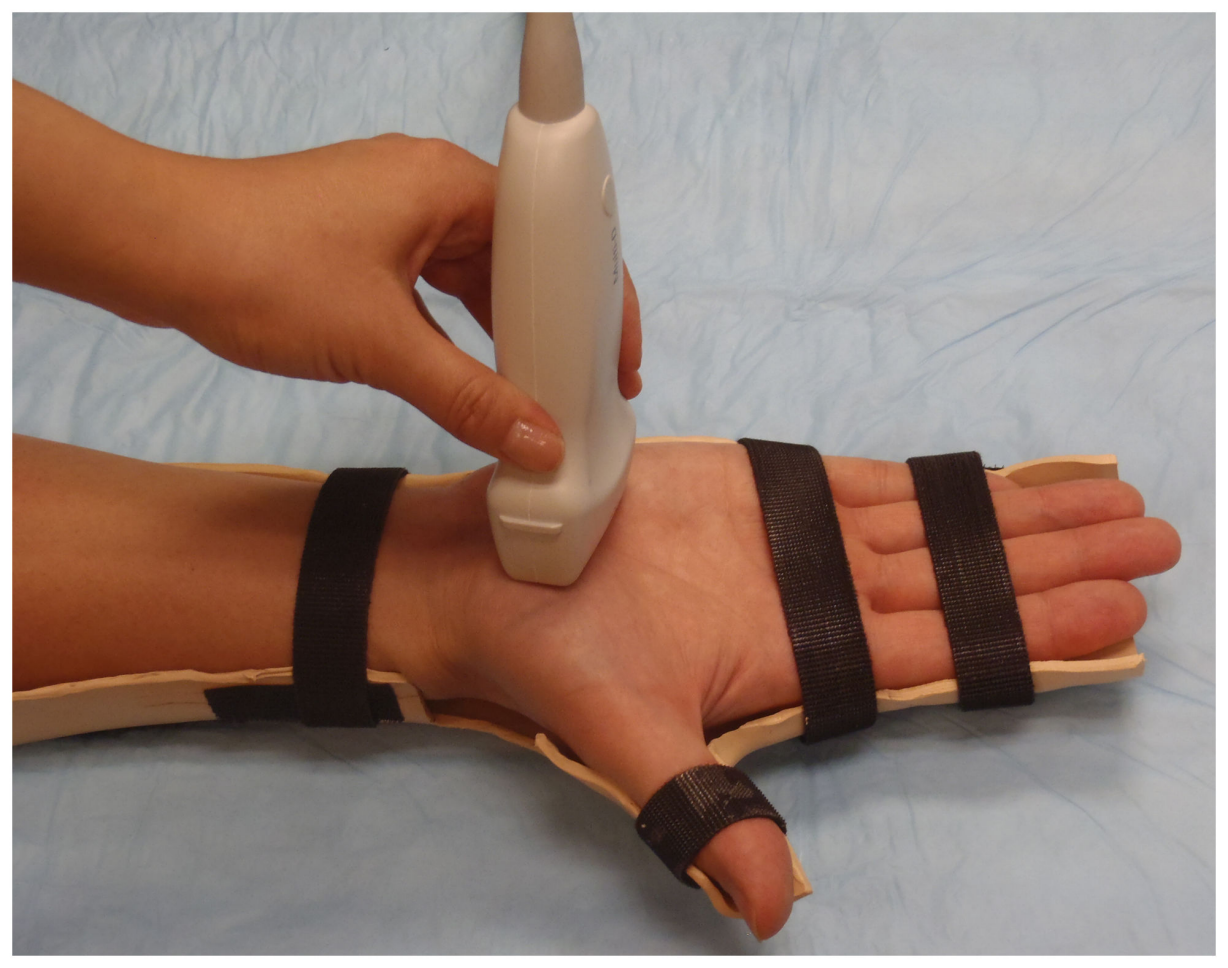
Positioning of the hand and ultrasound transducer for ARFI imaging. The right hand and forearm were stabilized in a custom-made splint using Velcro^®^ straps. The ultrasound transducer was placed at the distal level of the carpal tunnel.
